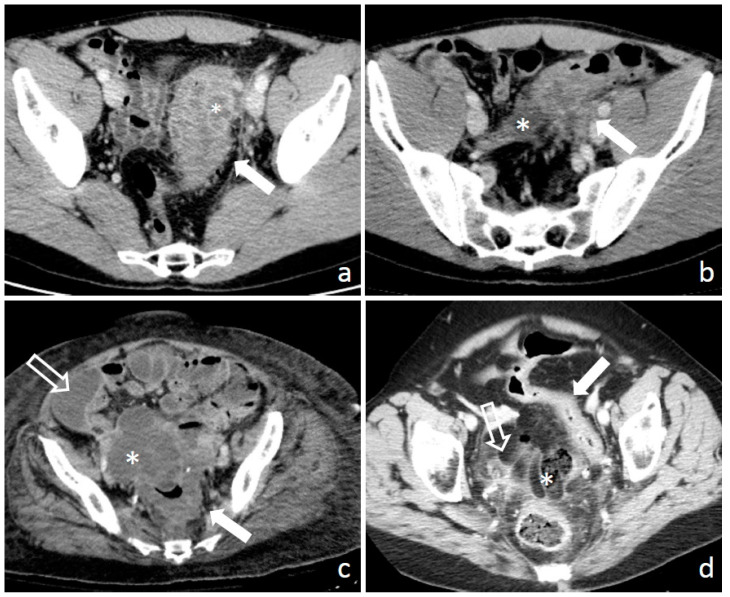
Modified Hinchey classification based on CT imaging: (a) Hinchey I—colonic wall thickening (arrow) with pericolic fat stranding and a localized abscess (*); (b) Hinchey II—colonic wall thickening (arrow), important fat stranding and a pelvic abscess (*); (c) Hinchey III—colonic wall thickening (arrow), with a pelvic abscess (*) and the presence of pus in the abdominal cavity (empty arrow) circumscribed by peritoneal thickening signs of purulent peritonitis; (d) Hinchey IV—colonic wall thickening (arrow) with intestinal perforation allowing feces into abdominal cavity (*) associated to fat and peritoneal stranding (empty arrow) signs of fecal peritonitis.
